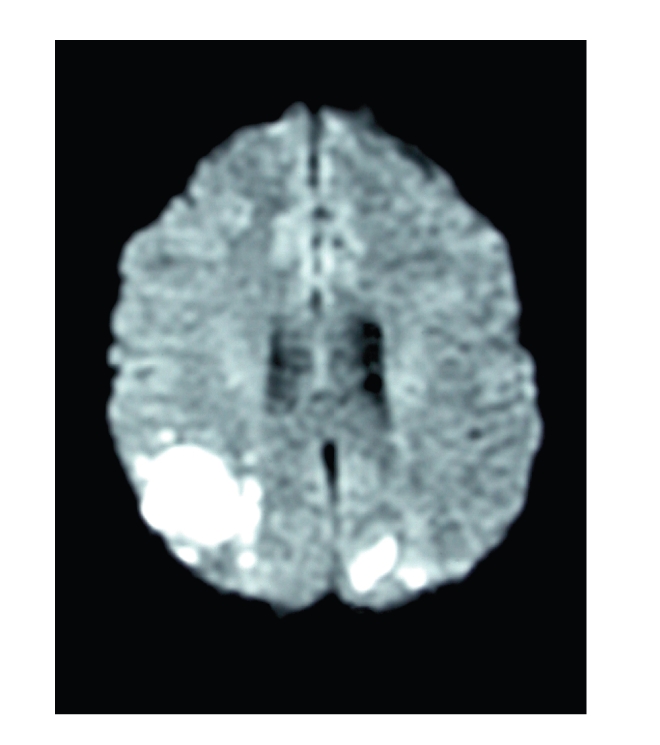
Diffusion tensor MRI emphasizing bilateral ischemia of parieto-occipital territories.
